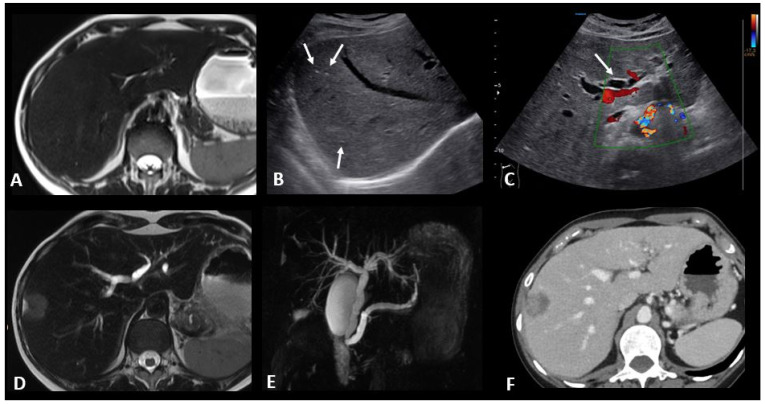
Immune-related hepatitis and cholangitis in a patient with an intestinal-type adenocarcinoma of the duodenum which demonstrated a high tumor burden mutation score (25.4 mut/Mb) and was treated in the first line with ipilimumab + nivolumab. One month after the start of treatment, there was an increase in ASAT, ALAT, and ALP to 2, 3, and 10 times the normal levels, respectively. EBV and CMV PCR in the blood, hepatitis serology, and HIV were negative. The abdominal ultrasound images in B-mode (B) and Doppler (C) show a heterogeneous liver parenchyma echogenicity with small echogenic foci (starry sky) (arrows figure (B)) and the appearance of intrahepatic bile duct dilatation (arrow figure (C) compared to the baseline T2 MR images (A). MR images with T2 (D) and cholangioMR (E) sequences demonstrate double bile duct and pancreatic duct dilatation with non-tumoral stenosis of the odi sphincter. Contrast-enhanced CT images acquired 2 months after the stop of immunotherapy (F) show a nearly complete regression of the dilation of the intrahepatic bile ducts.
